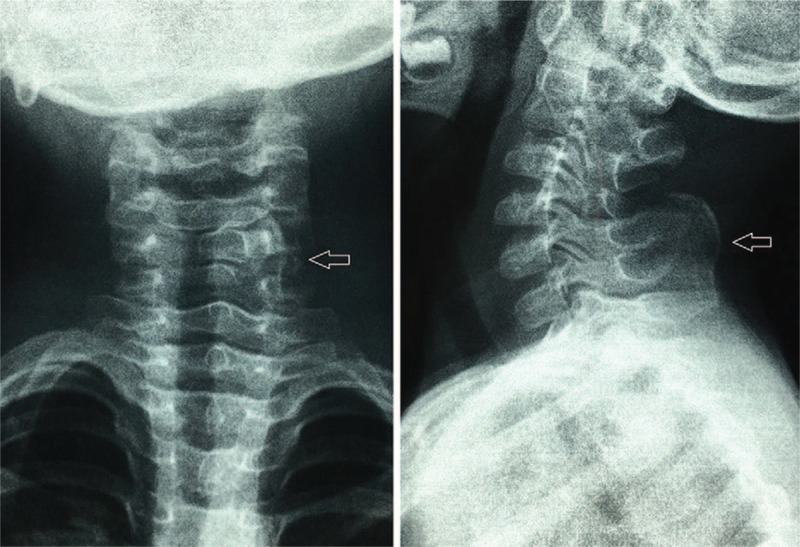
Plain radiographs of the cervical spine demonstrated an anomalous bone located posterior to the C5 vertebrae (arrow).
